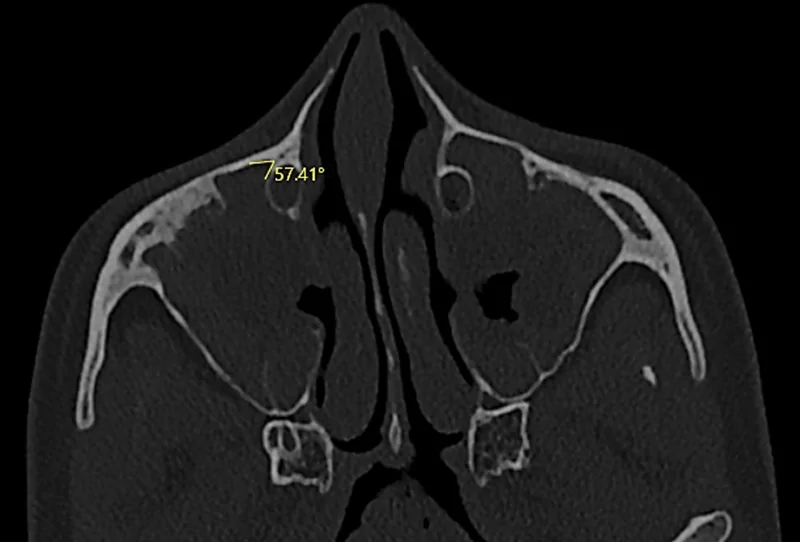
Computed tomography scan showing low grade feasibility of the transnasal prelacrimal recess approach (TPRA) in right chronic rhinosinusitis type Ia, with the yellow line representing the angle of the TPRA.
